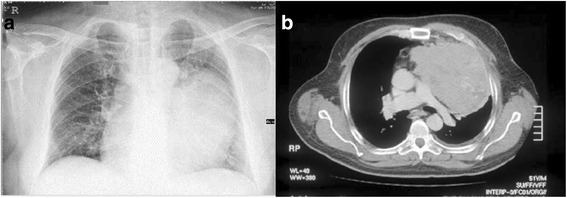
Radiological findings of mediastinal mass. a Chest X-ray showing large left mediastinal mass. b Computed tomography of chest with contrast showing left-sided radiodense mass with calcifications
